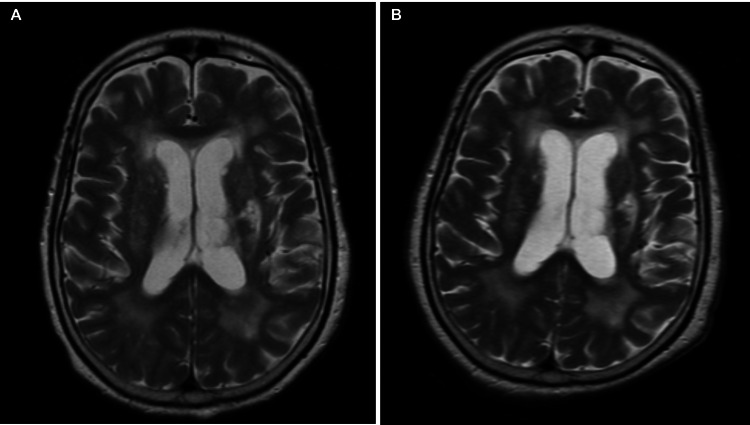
MRI brain without contrast MRI showed numerous punctate focal lesions on T2 hyperintensity involving the gray-white matter junction of bilateral cerebral hemispheres and within the left middle cerebellar peduncle (A), which were new compared to admission MRI (B).
